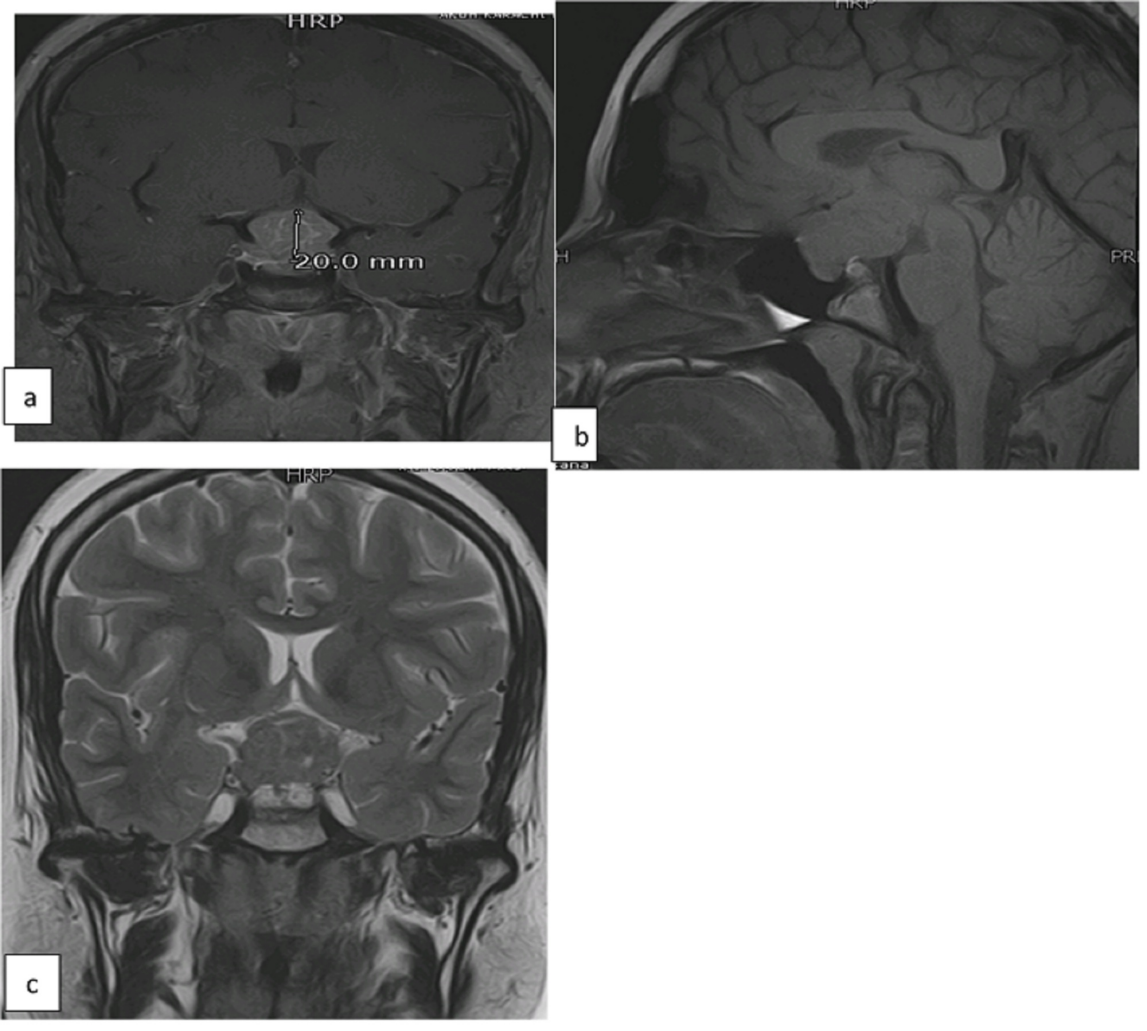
Preoperative MRI pituitary gland with (a) T1 coronal, (b) T1 sagittal, and (c) T2 coronal images showing a lobulated mass in the sellar region with suprasellar extension. It is iso to hyperintense on T1W image and isointense on T2W image.
